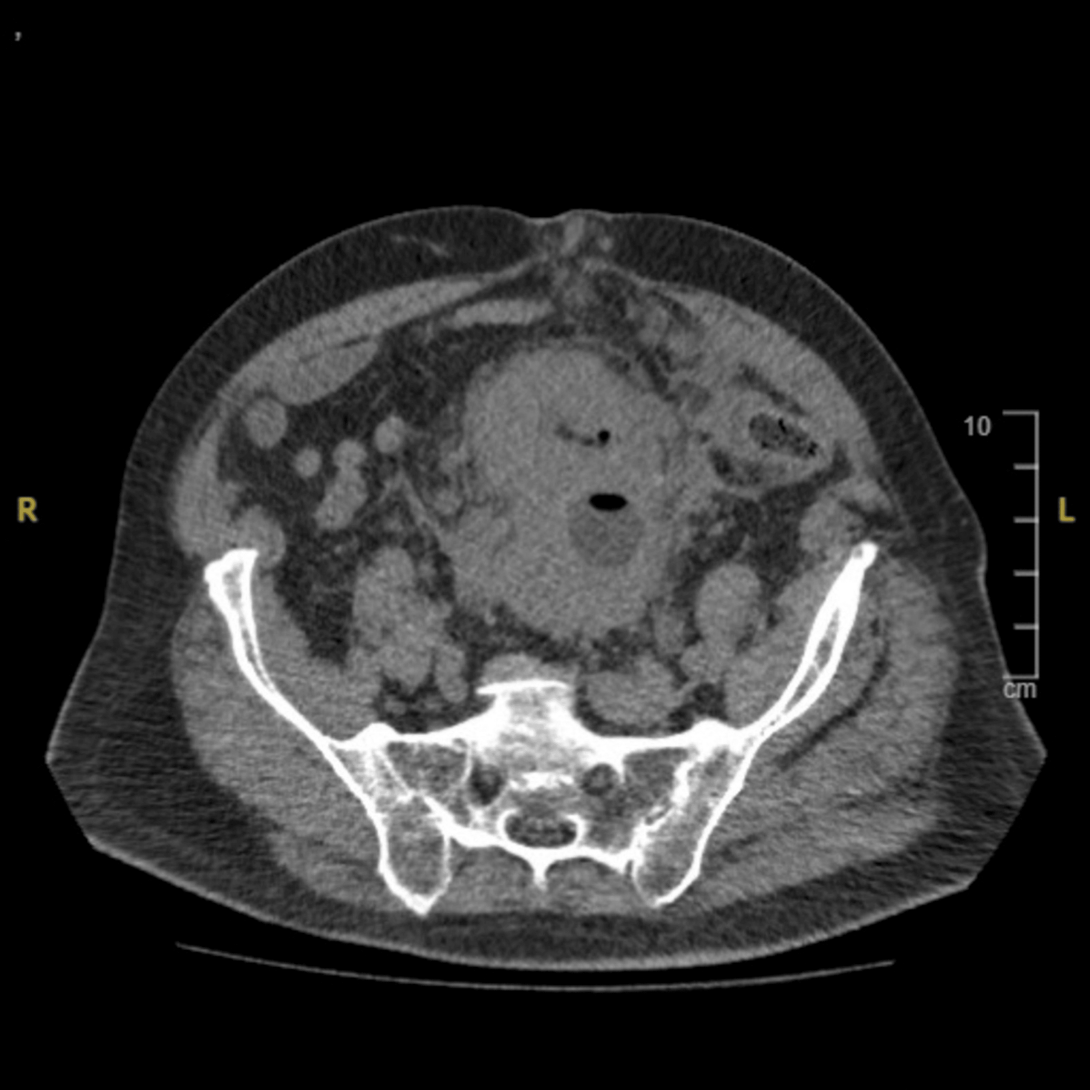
Axial view of abdominopelvic computed tomography showing a large bladder tumor.
